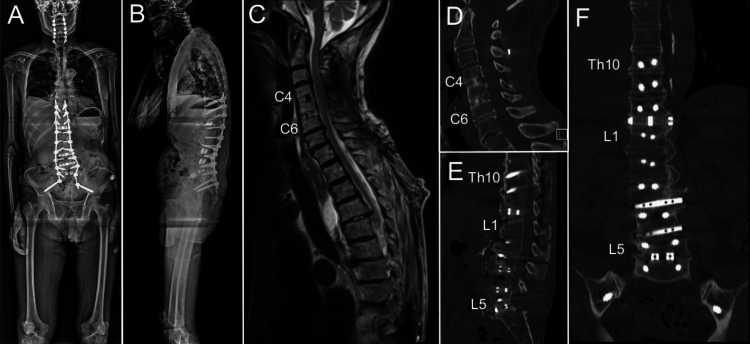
Radiographic images after the second operation Oblique and posterior lumbar intervertebral fixations concurrently performed with posterior long fusion from Th10 to the ilium. The Cobb angle and sagittal vertical axis were 15.9° and 50.5mm, respectively (A: lateral projection; B: anteroposterior projection). Sagittal spinal magnetic resonance imaging five months after the second surgery reveals resolution of the retropharyngeal abscess, epidural abscess, spondylodiscitis, and pyogenic spondylitis (C). Achievement of bone fusion is identified on computed tomography images six months after the second surgery (D-F)
